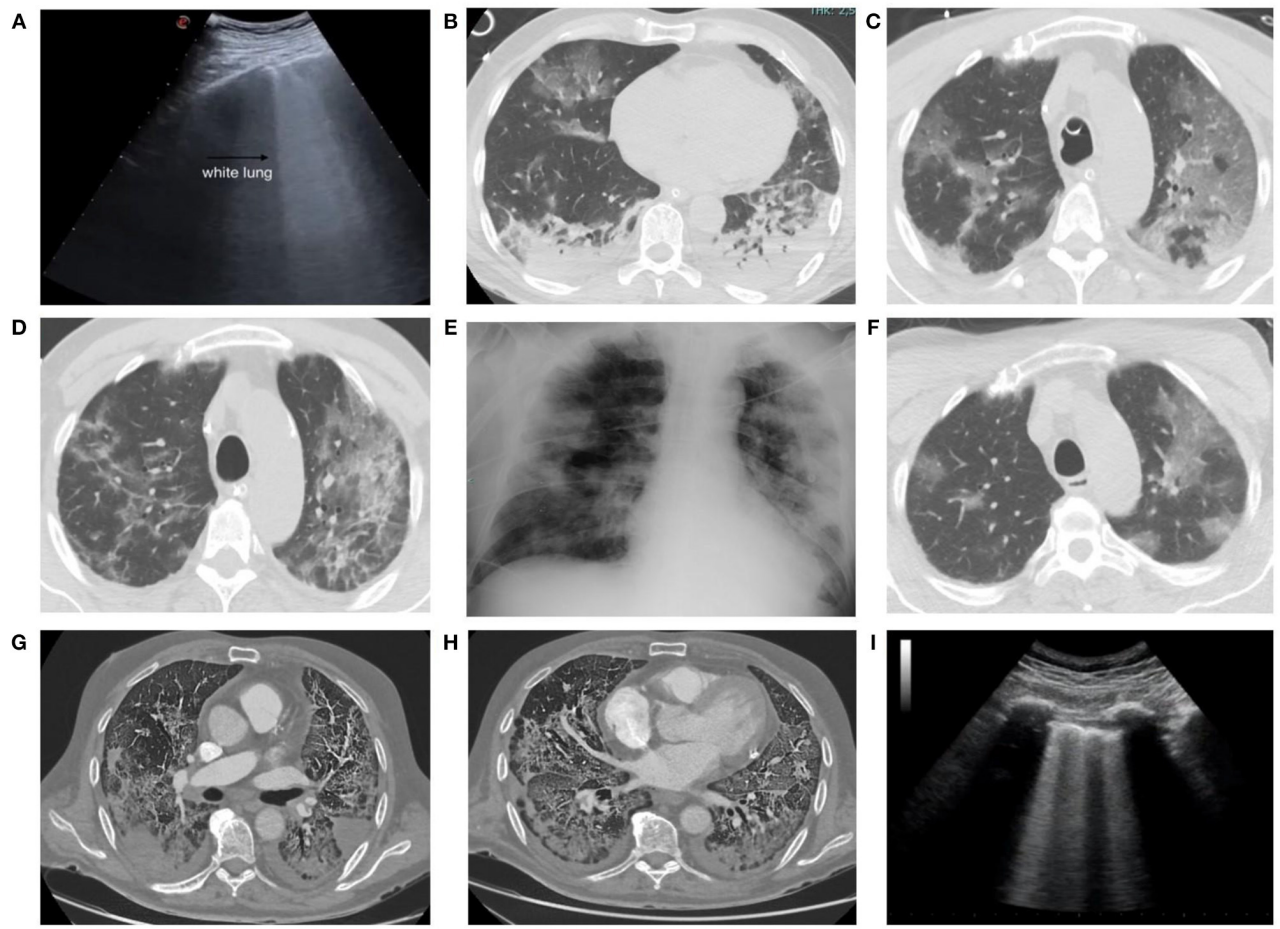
A 55-year-old man admitted to our emergency department with signs and symptoms of respiratory infection underwent chest ultrasound (US) (A), showing white lung areas, then confirmed at computed tomography (CT) examination (B) which showed multiple bilateral subpleural areas of ground glass opacity (GGO) in the upper lobes. CT scans obtained 48 h after admission showed the multiple GGO areas increased in number and extension (C) and the development of pulmonary consolidations in the basal posterior and lateral segments of both lower lobes (D). Chest x-ray obtained 9 days after admission (E) showed multiple coalescent pulmonary consolidations involving both lungs, mainly in the periphery. CT scans obtained 13 days after admission showed the GGOs in the upper lobes now partly regressed (F). CT scan in a 75-year-old man, with severe lung involvement, secondary to SARS-CoV-2 infection, showing pulmonary thromboembolic disease involving segmental arteries in the left (G) and right (H) lower lobes with triangular shaped subpleural consolidations (pulmonary infarcts). A 68-year-old man admitted to our emergency department with signs and symptoms of respiratory infection then resulted Covid+. Chest US showed an interstitial syndrome, characterized by more than three B lines per longitudinal field (I).
